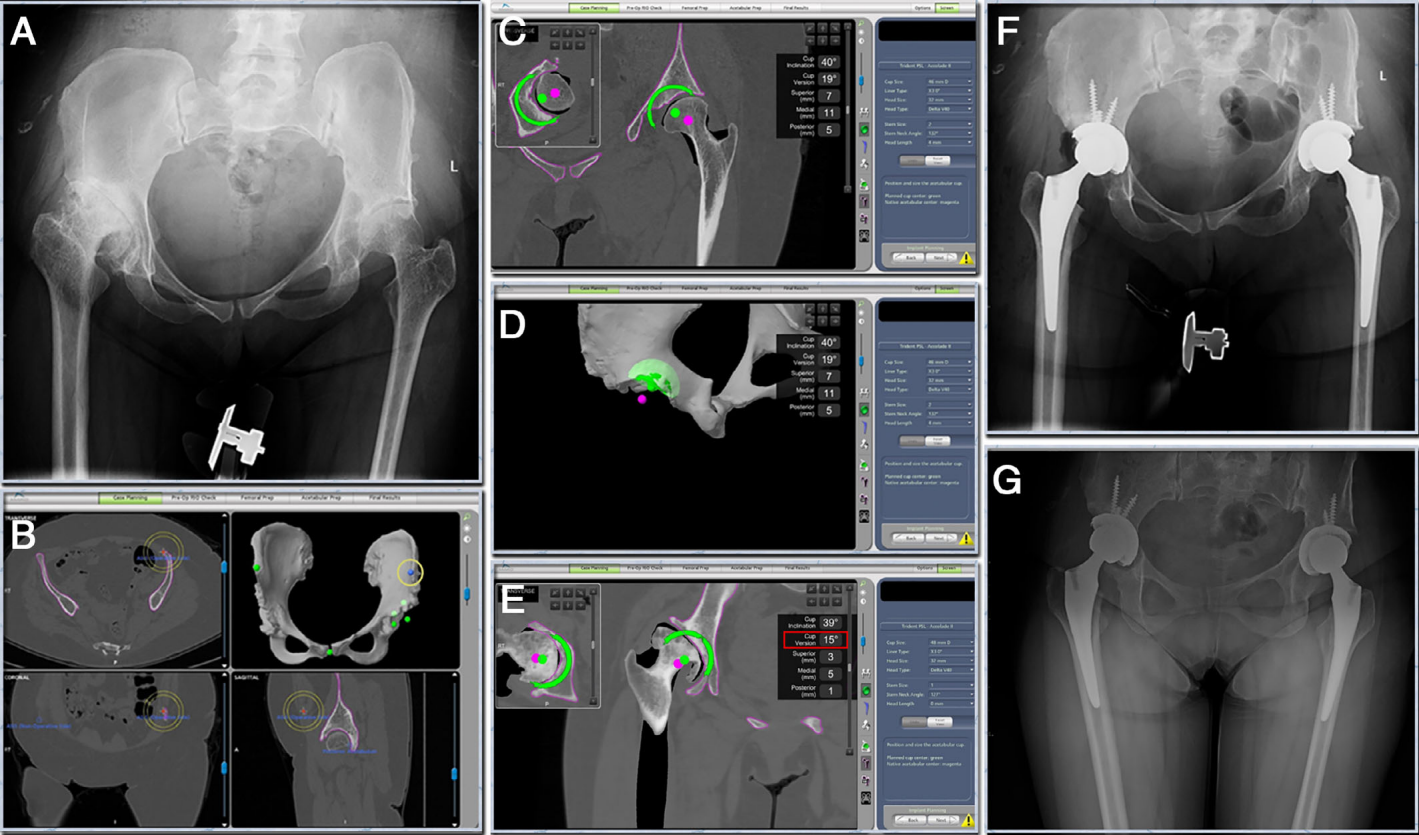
(A) Pre‐operative radiographs. The patient suffered from bilateral DDH. (B) & (C) & (D) & (E) CT based pre‐operative planning. Left ASIS was absent due to previous osteotomy (yellow circle). The position of the left ASIS reference point was carefully adjusted to make the pelvis axis as horizontal as possible. The cups were planned to reconstruct with the high hip center technique. The right cup anteversion was planned to be lower than usual (red square). (F) The post‐operative X‐rays showed that the robotic arm assisted THA had achieved the surgery plan. (G) Radiograph 3‐months post‐operative showed no change in the position of the prosthesis.
